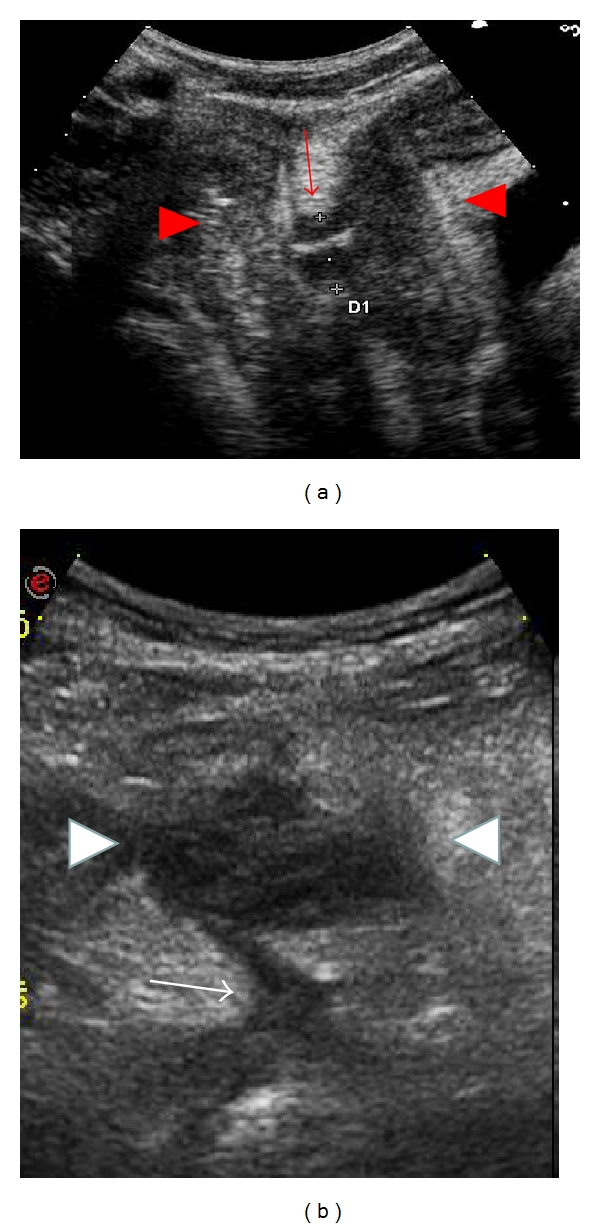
Bowel ultrasound in a 22-year-old female with ileocolonic Crohn's disease. In (a) red arrow indicates enteroenteric fistula (defined as a hypoechoic track with a hyperechoic content) between diseased ileal loops with bowel wall thickness (red arrowheads). In (b) a small abscess (white arrowheads identify a roundish anechoic lesions with irregular walls, presenting internal echoes) with fistula (white arrow) was identified in the same patient.
